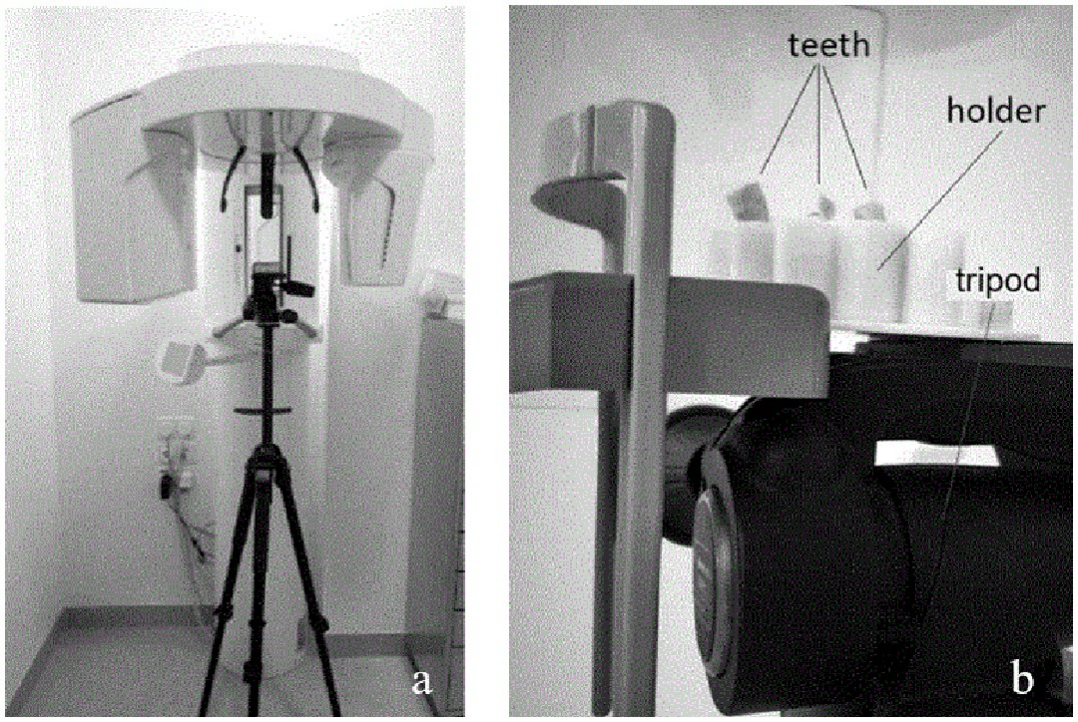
Experimental setup to create CBCT images. (a) Overview image of the CBCT device (Orthophos XG 3D, Dentsply Sirona; York, PA, USA) and tripod. (b) Detailed image of the tripod with plastic holder and teeth.
